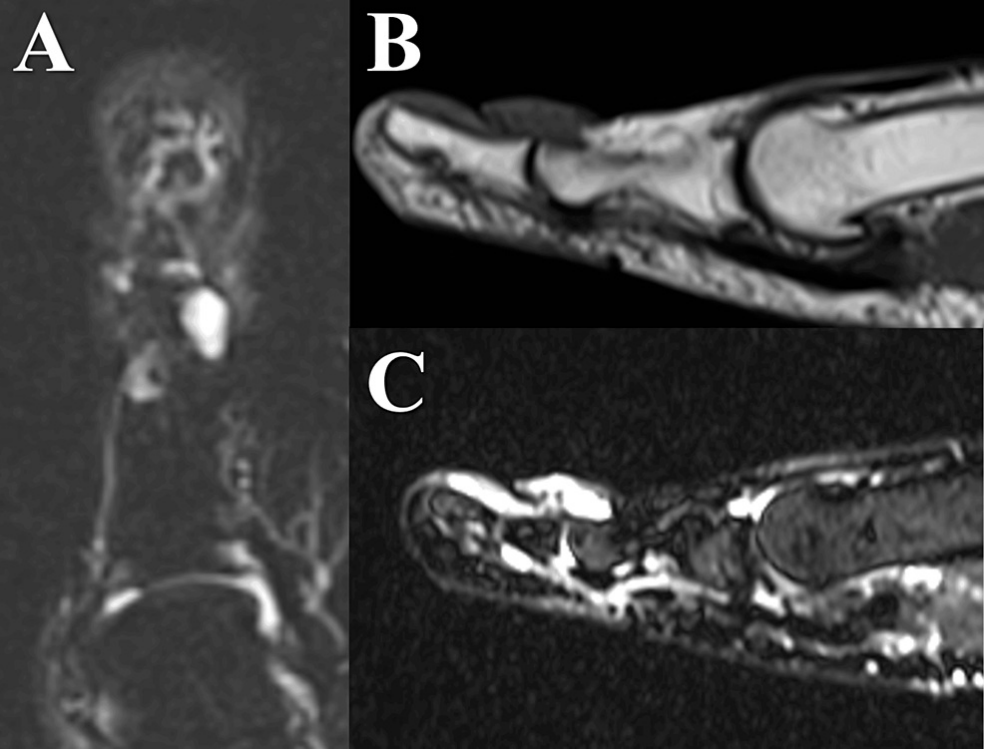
Findings on MRI (Case 2) A solitary cyst was found on the dorsal aspect of the interphalangeal joint. T1-weighted (B) and short tau inversion recovery (A-C) sequences showed fluid accumulations within the joint and the tendon sheath of FHL. MRI: magnetic resonance imaging, FHL: flexor hallucis longus
